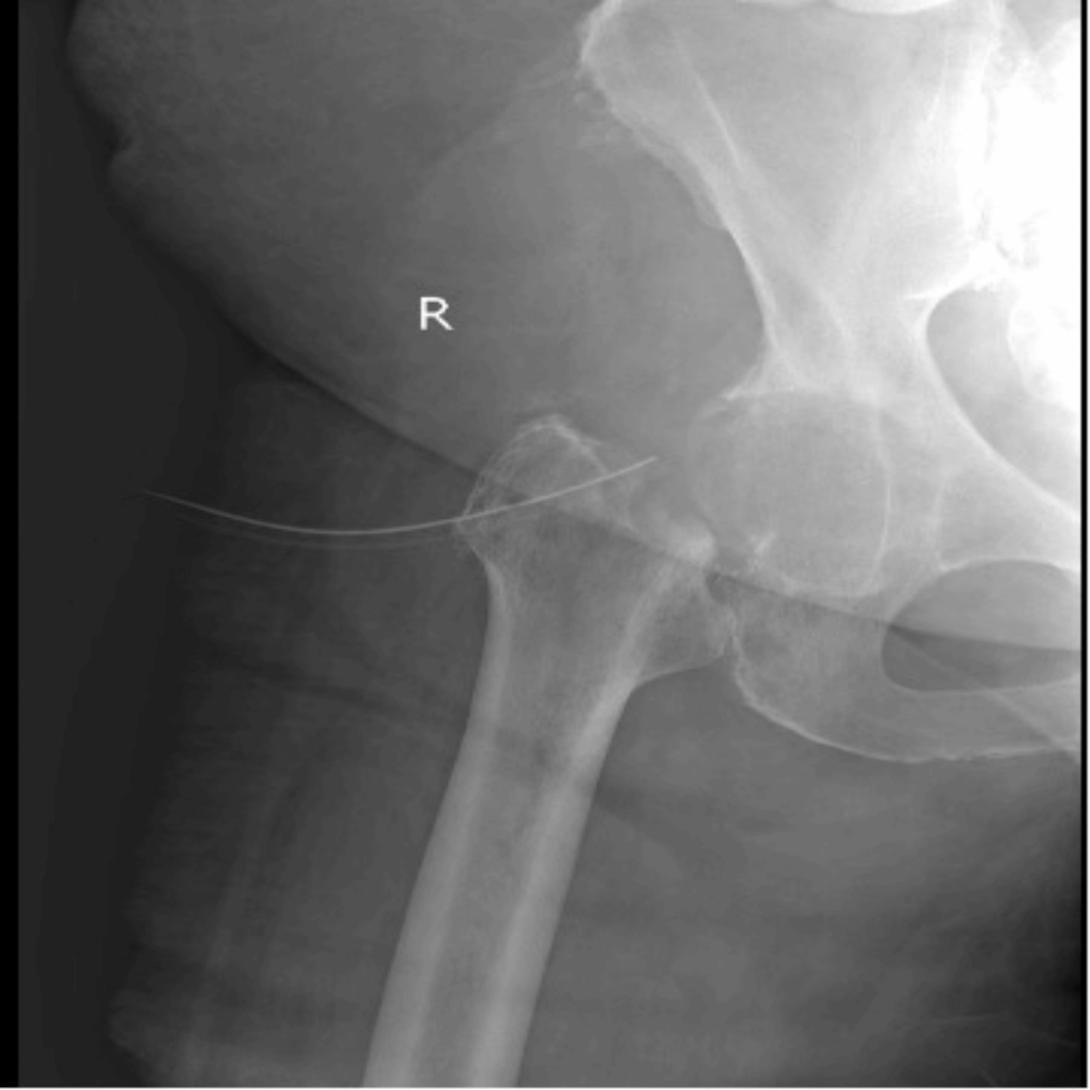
Plain radiograph after additional debridement and spacer removal
